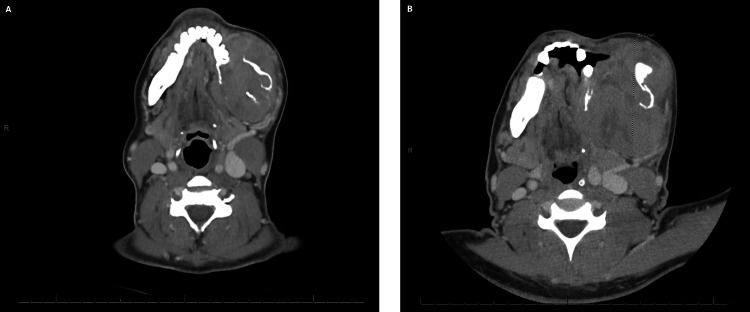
Axial computed tomography (CT) with contrast of an expansile mass centered in the left mandibular body A) The mass during a previous hospital visit one month prior measuring 5.7 x 6.1 x 8.2 cm (AP by TV by CC); B) The mass at time of presentation measuring 8.1 x 5.6 x 9.4 cm (AP by TV by CC) AP: anteroposterior; TV: transverse; CC: craniocaudal
